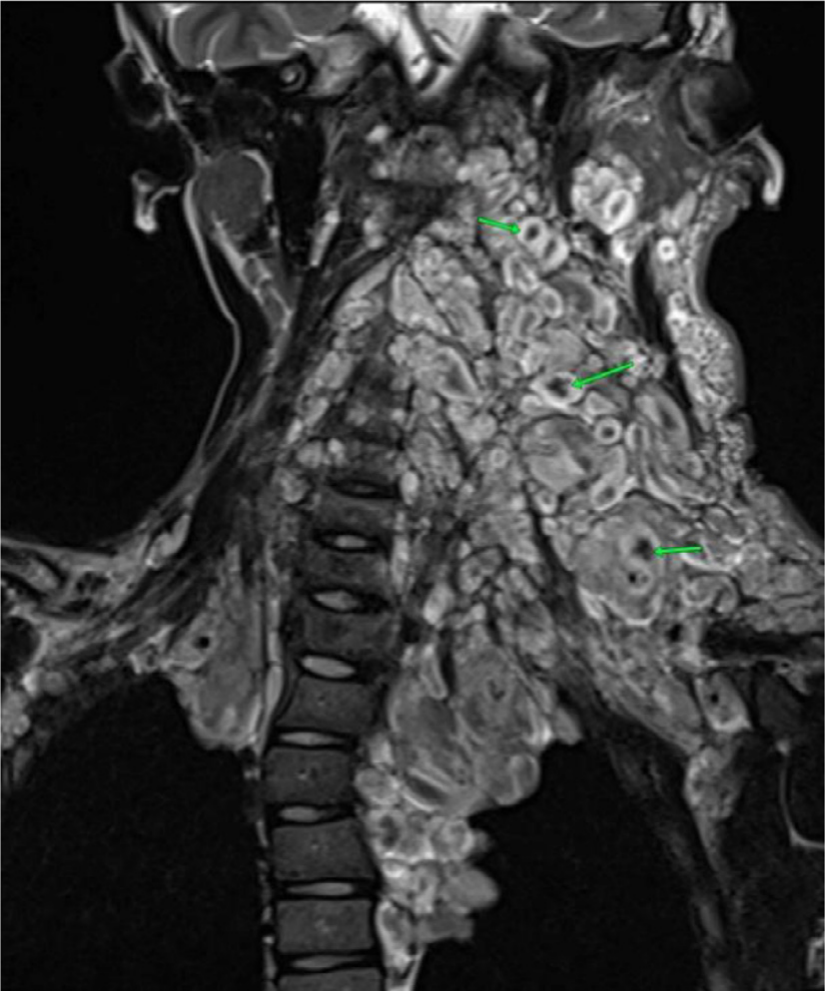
Cervical MRI of the patient with plexiform neurofibromaTarget sign on T2 weight image.
